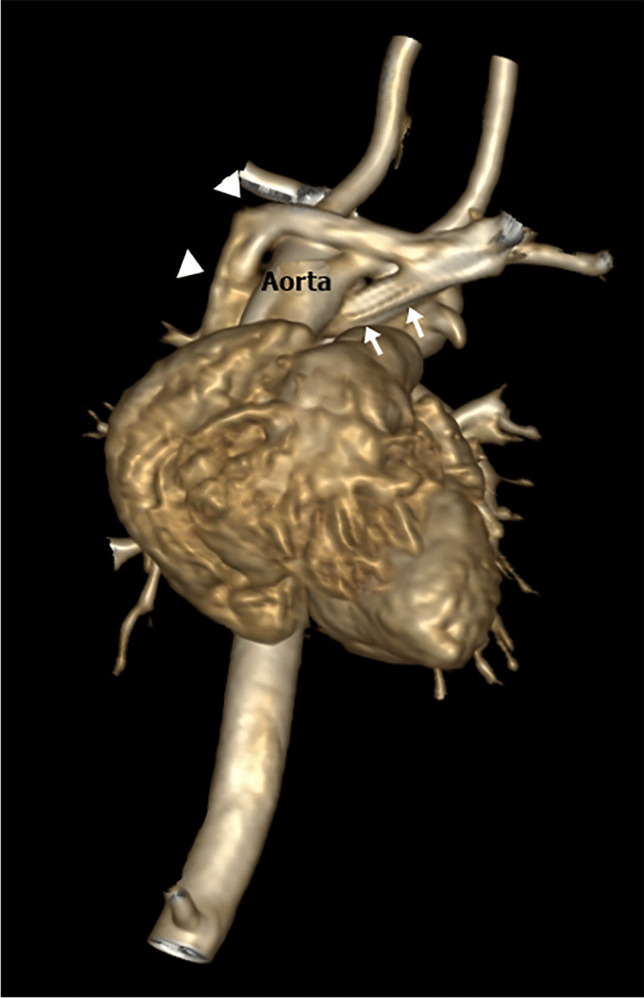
A double left brachiocephalic vein with no other anomalies in a 1-week-old girl. A volume-rendered computed tomography image shows one left brachiocephalic vein (*arrowheads*) passing anteriorly to the aorta and the other left brachiocephalic vein (*arrows*) passing posterior to the aorta
